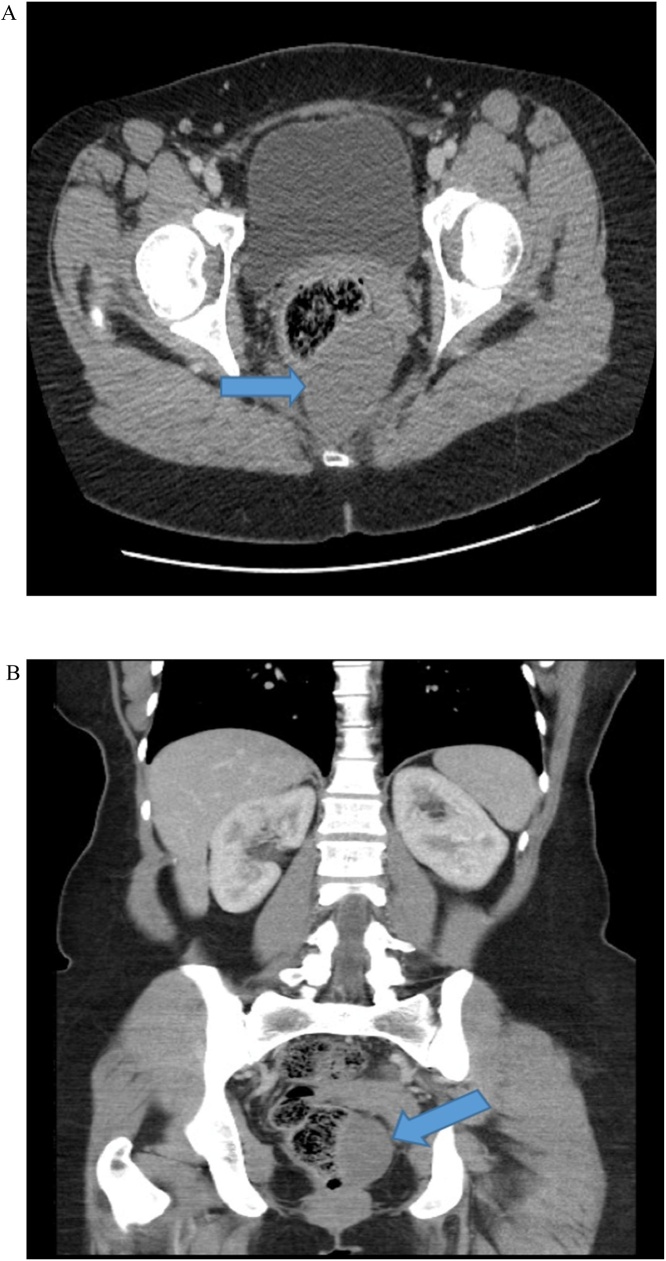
A: CT scan of the abdomen revealed a unilocular non-enhancing low-attenuation mass at the retrorectum measuring 5.4 × 4.6 cm. B: Coronal view of the same image.
